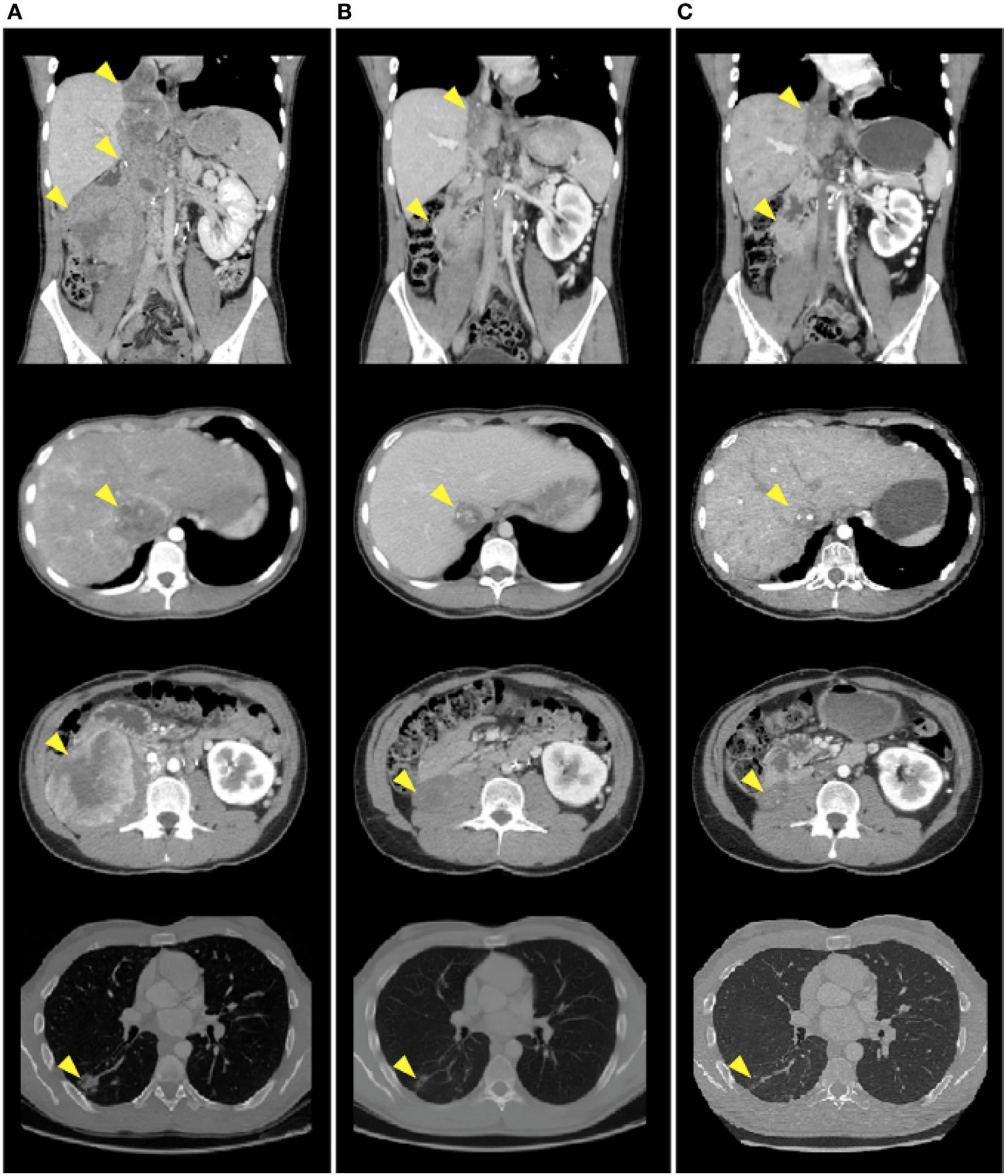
CT scan images demonstrate a decrease in size of surgical site nodules, inferior vena cava tumor thrombus, paraaortic lymph node metastasis, and lung nodules after eight and fifteen infusions of nivolumab. (A) Before nivolumab therapy initiation. (B) After eight infusions of nivolumab. (C) After fifteen infusions of nivolumab.
